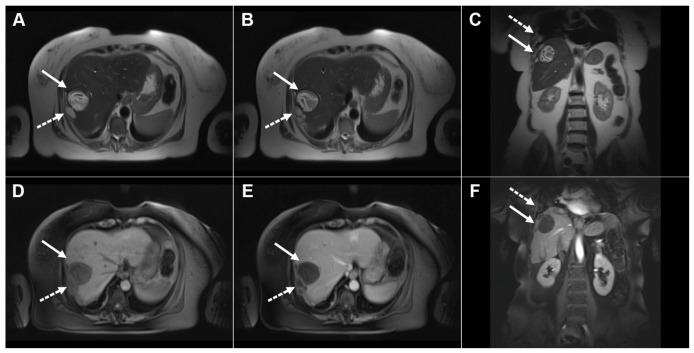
Complicated hydatid cyst in a 73-year-old female. Axial T2-weighted images (A,B) demonstrate a cystic lesion (*arrows*) with a detached germinal membrane and a small rupture, resulting in extrahepatic extension (*dotted arrows*). Coronal T2-weighted image (C) shows transdiaphragmatic extension of the cyst into the thoracic cavity (*arrow*, *dotted arrow*). The liver lesion (*arrow*) and extrahepatic part (*dotted arrow*) are hypointense in the T1-weighted fat-suppressed image (D), without enhancement in the portovenous phase image (E). Coronal post-contrast image (F) demonstrates absent enhancement of both the liver lesion (*arrow*) and the transdiaphragmatic cystic extension (*dotted arrow*).
